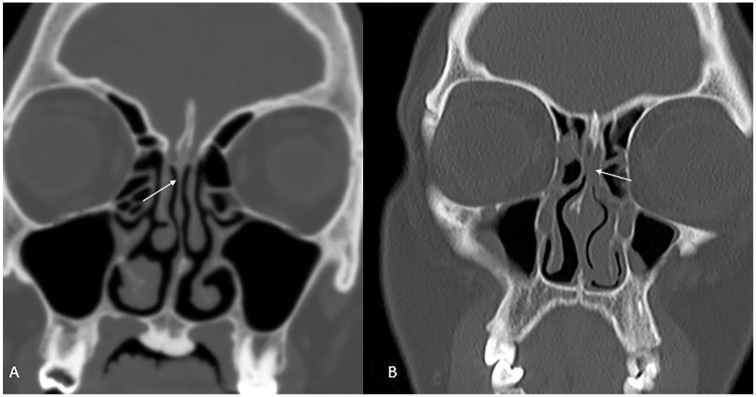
Olfactory recess grades. Coronal CT scan images demonstrates an aerated
